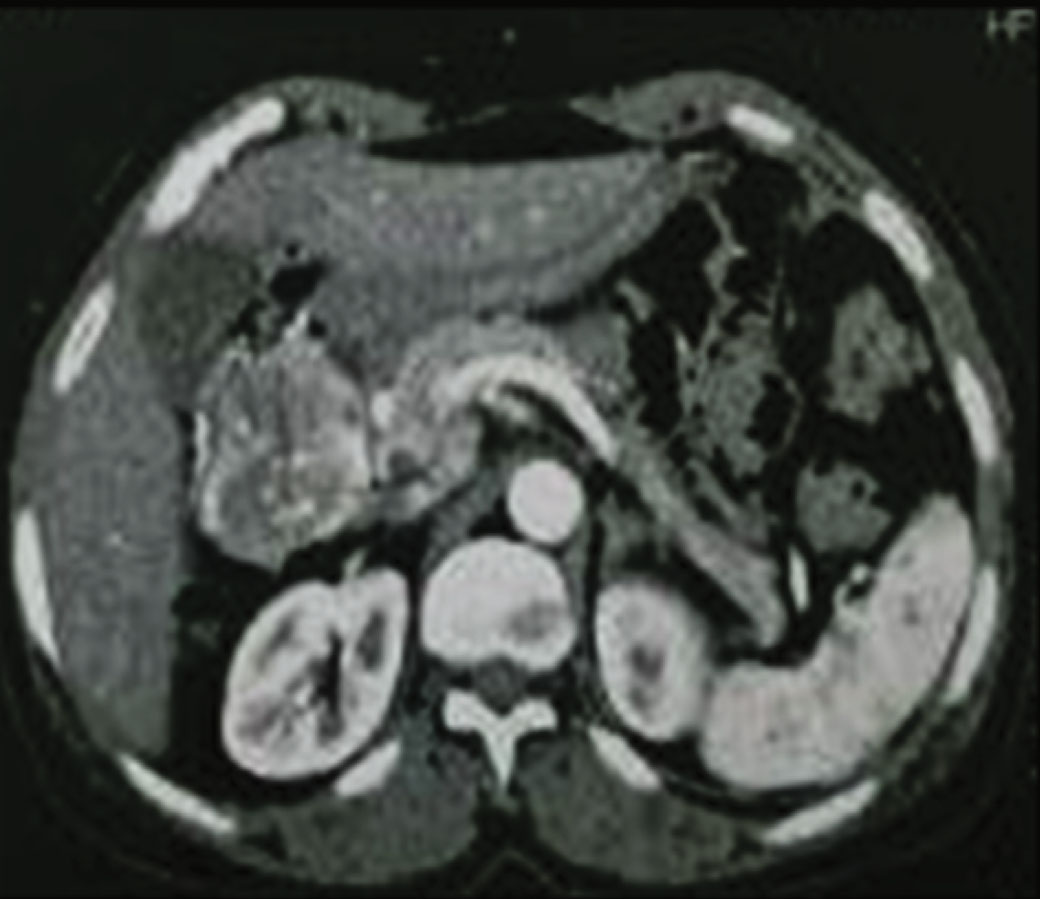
Abdominal CT enhanced scan shows a 5 cm exophytic duodenum mass, revealing a stromal tumor.
